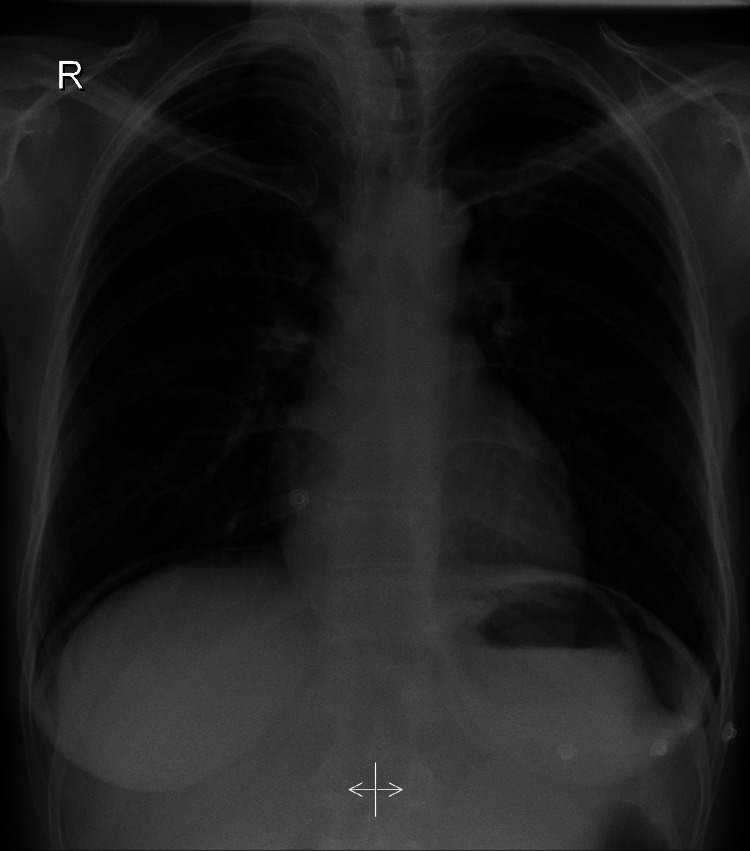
Chest radiograph showing air under the diaphragm bilaterally
